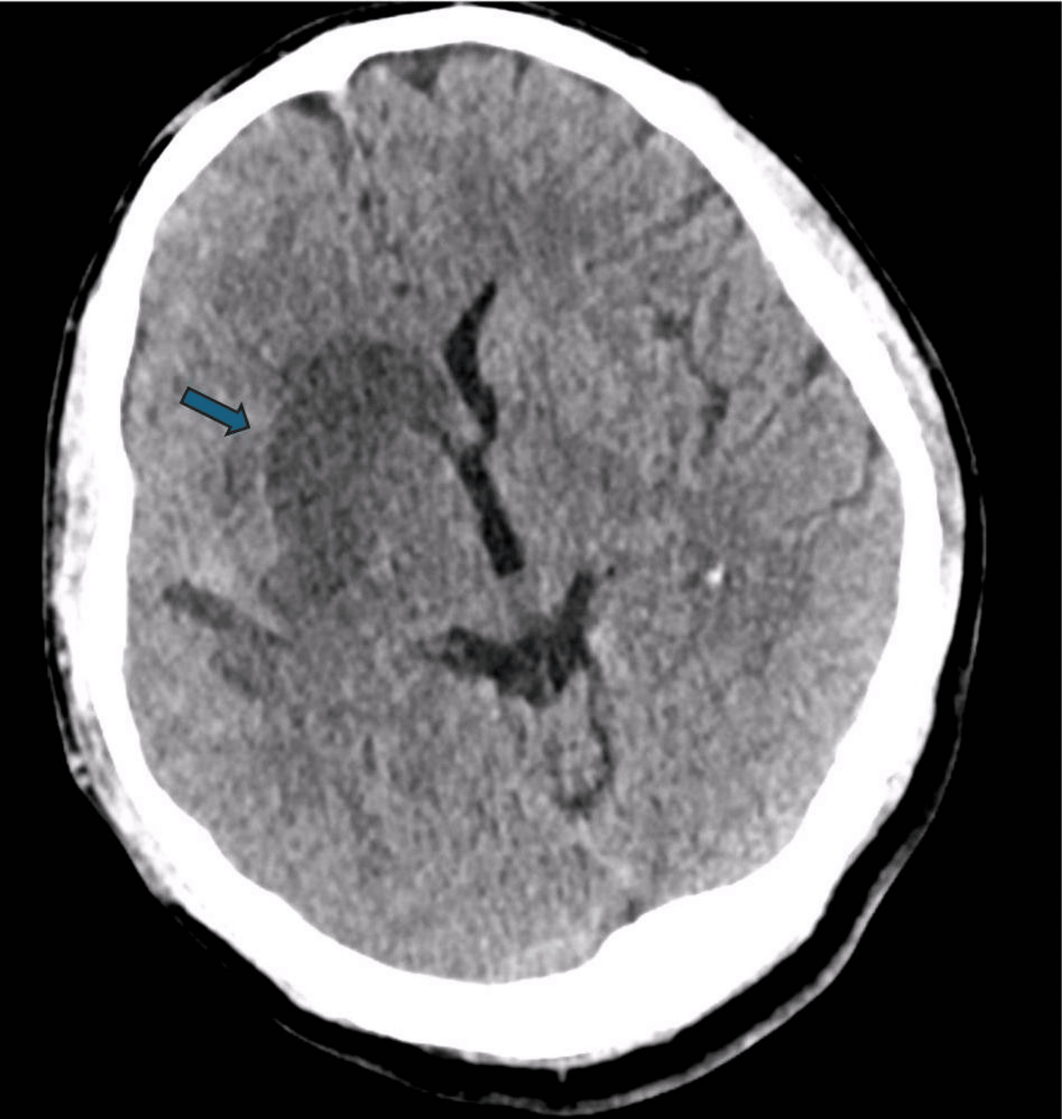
A CT scan of the head obtained 24 hours post thrombectomy showed an established infarct within the right basal ganglia and opercular region, similar to the previous CT from 09/06/25. No convincing acute haemorrhage was noted.
